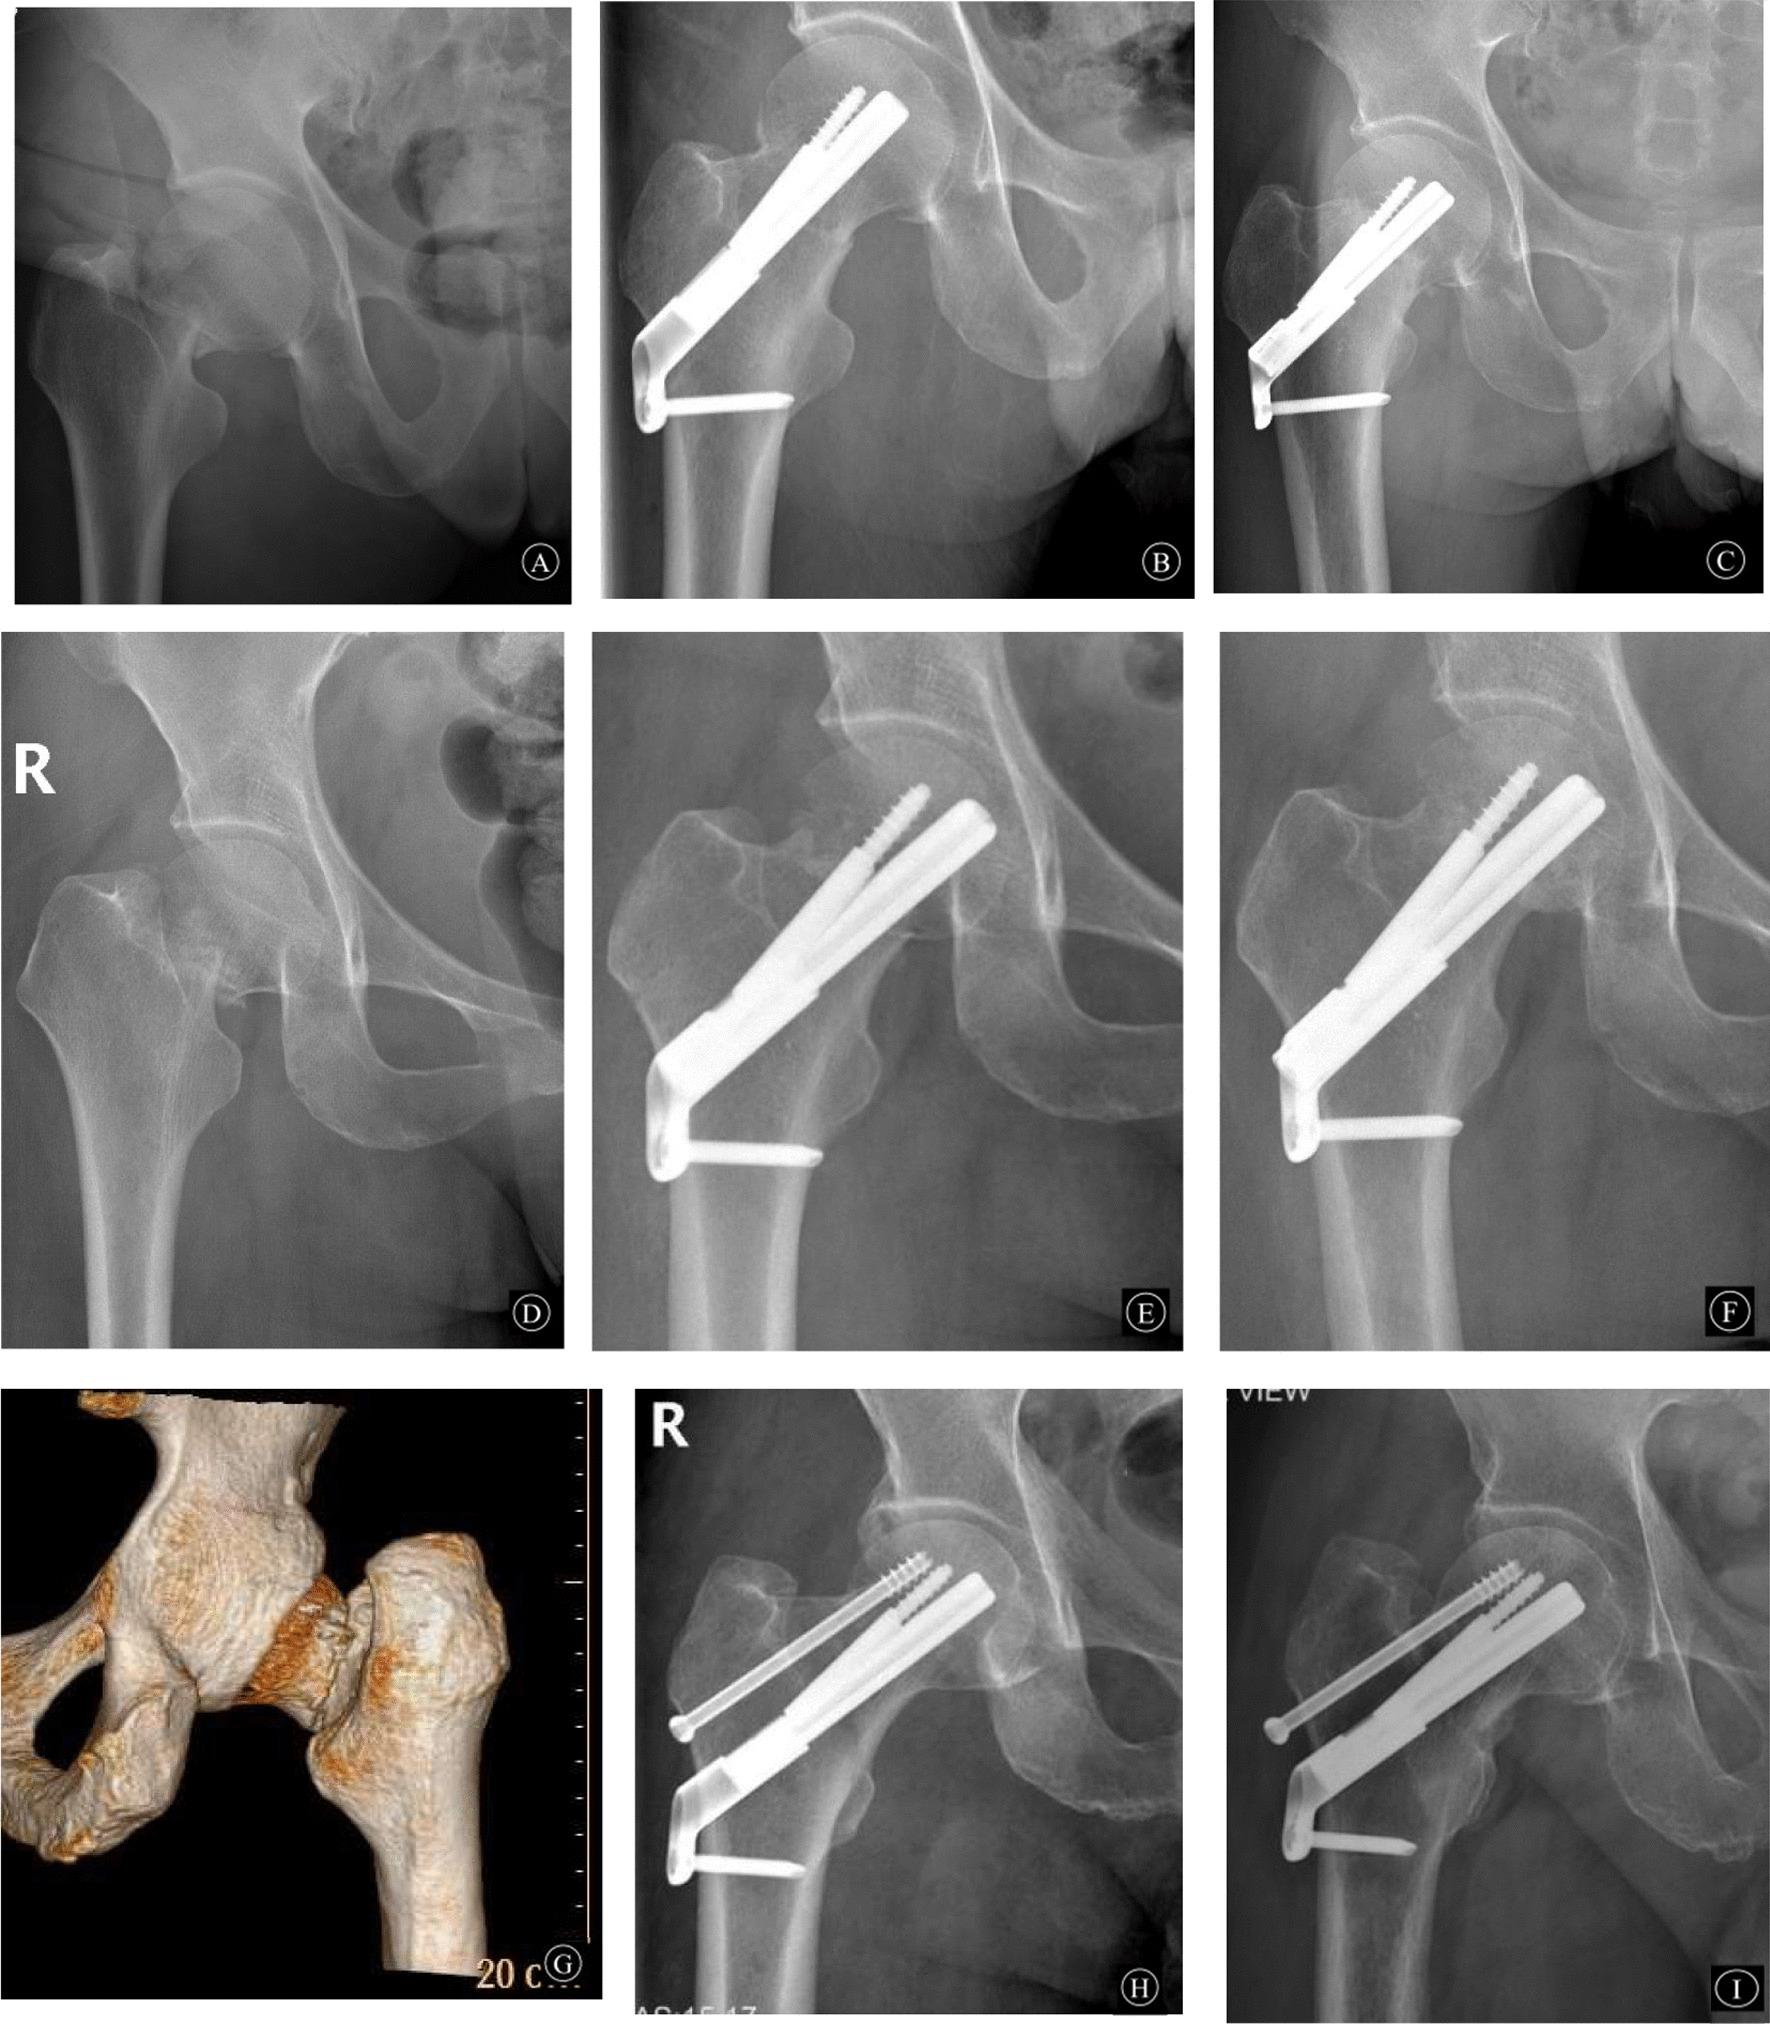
A, B, C are 53-year-old men, A preoperative hip orthogonal position shows right femoral neck fracture (Garden type IV), B postoperative hip orthogonal position FNS sliding space is not limited (20 mm); C 6 months postoperative femoral neck is severely shortened, FNS still has about 5 mm sliding space. D, E, F are 58-year-old women, D preoperative hip orthogonal position shows right femoral neck fracture (Garden type IV), E postoperative hip orthogonal position FNS sliding space restricts sliding space (about 5 mm), F 6 months postoperative femoral neck mild shortening (shortening < 5 mm). G, H, and I were 54-year-old men; G's preoperative CT 3D reconstruction of the hip showed posterior medial cortical comminution; H's postoperative hip was in an orthogonal position near anatomic reset; I's right hip was in an orthogonal position 6 months postoperatively with severe shortening of the femoral neck (< 10 mm of shortening)
